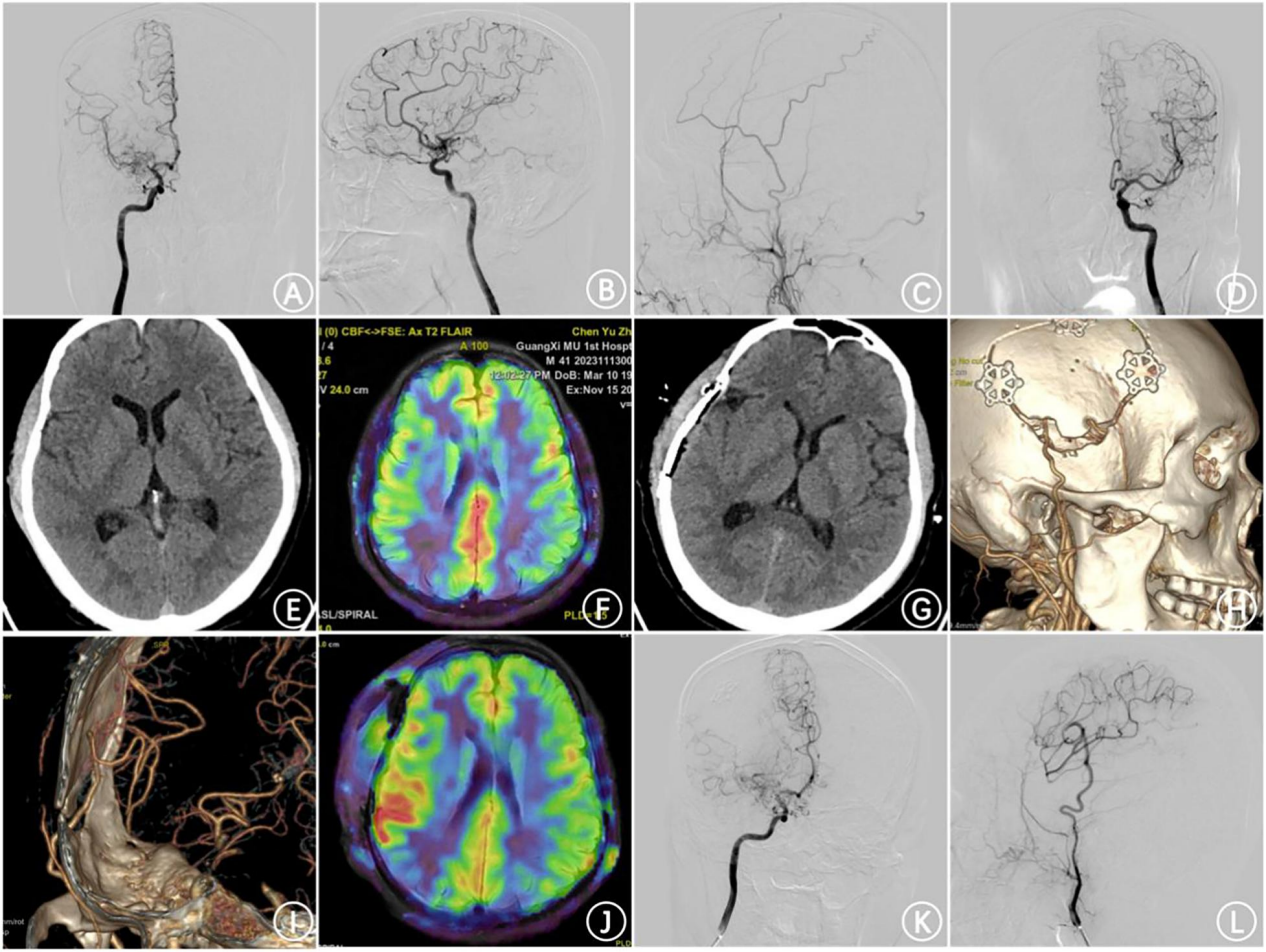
Imaging follow-up of cases of STA-MCA + EMS combined blood flow reconstruction surgery (A,B) preoperative DSA indicated stenosis at the terminal end of the right internal carotid artery and smoke vessel formation; (C) preoperative DSA lateral angiography of the left external carotid artery showed no obvious compensation; (D) preoperative DSA indicated that no obvious abnormalities were found in the left internal carotid artery angiography; (E) preoperative CT plain scan showed no obvious abnormalities; (F) preoperative perfusion imaging indicated a decrease in CBF in the right hemisphere; (G) no obvious abnormalities were found in the CT re-examination on the first day after the operation; (H,I) one week after the operation, the reexamination of CTA indicated that the STA-MCA anastomosis was unobstructed; (J) one week after the operation, perfusion imaging indicated that the CBF in the right hemisphere was higher than that before the operation; (K,L) the DSA reexamination half a year after the operation indicated that the STA was significantly thickened and supplied blood to the MCA.
